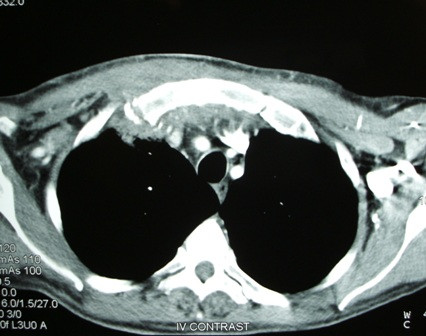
Computed tomography scan showing inflammatory changes behind the right sternoclavicular joint with small pockets of air behind the upper sternum.
